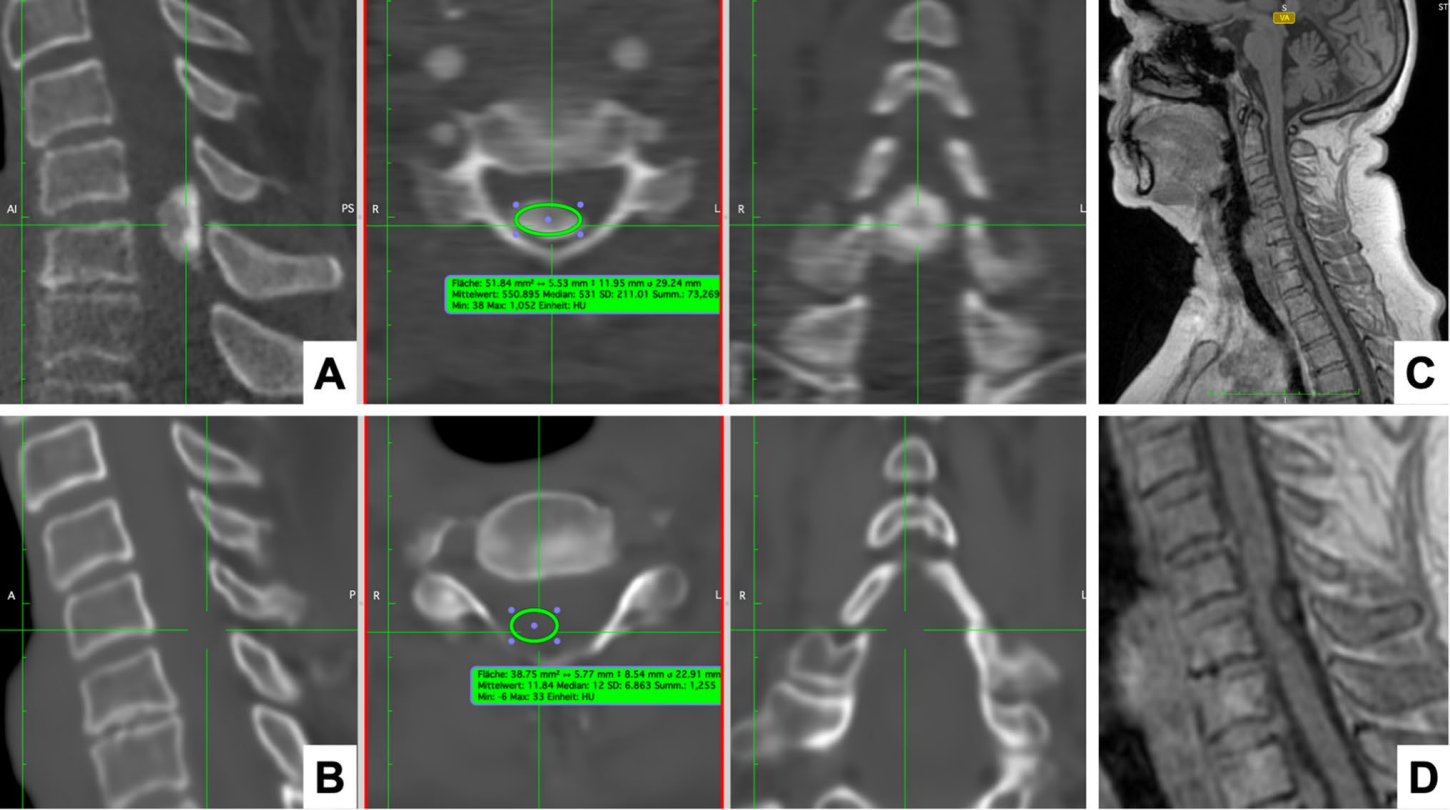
Case vignette of a 71-year-old female patient with a densely calcified intradural meningioma (mean HUs 551). A In the conventional CT image, the densely calcified tumor is well visible, the dimensions measured were 13.8 mm (axial) x 16 mm (sagittal) and mean HUs of 550.8 in the axial view. B On the synthetic CT image, the tumor was not visible (0 × 0 mm), and the mean HUs of the tumor region were 11.8. C-D On the source image (3D radiofrequency-spoiled T1-weighted multiple gradient echo (T1w-MGE) sequence), the tumor can be seen
